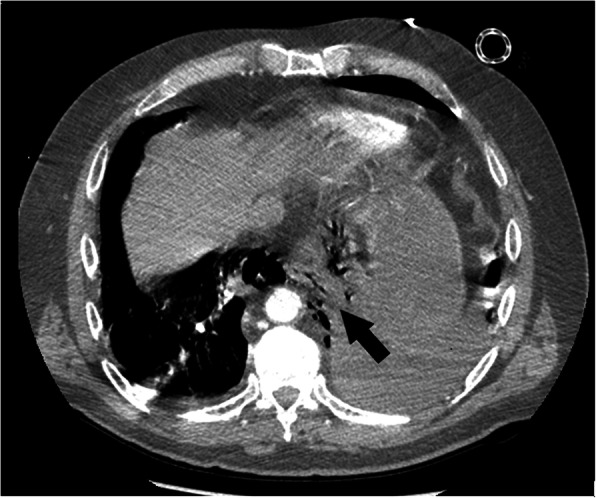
Computed tomography chest revealed gas bubbles laterally to the gastro-esophageal junction (arrow). Axial view
